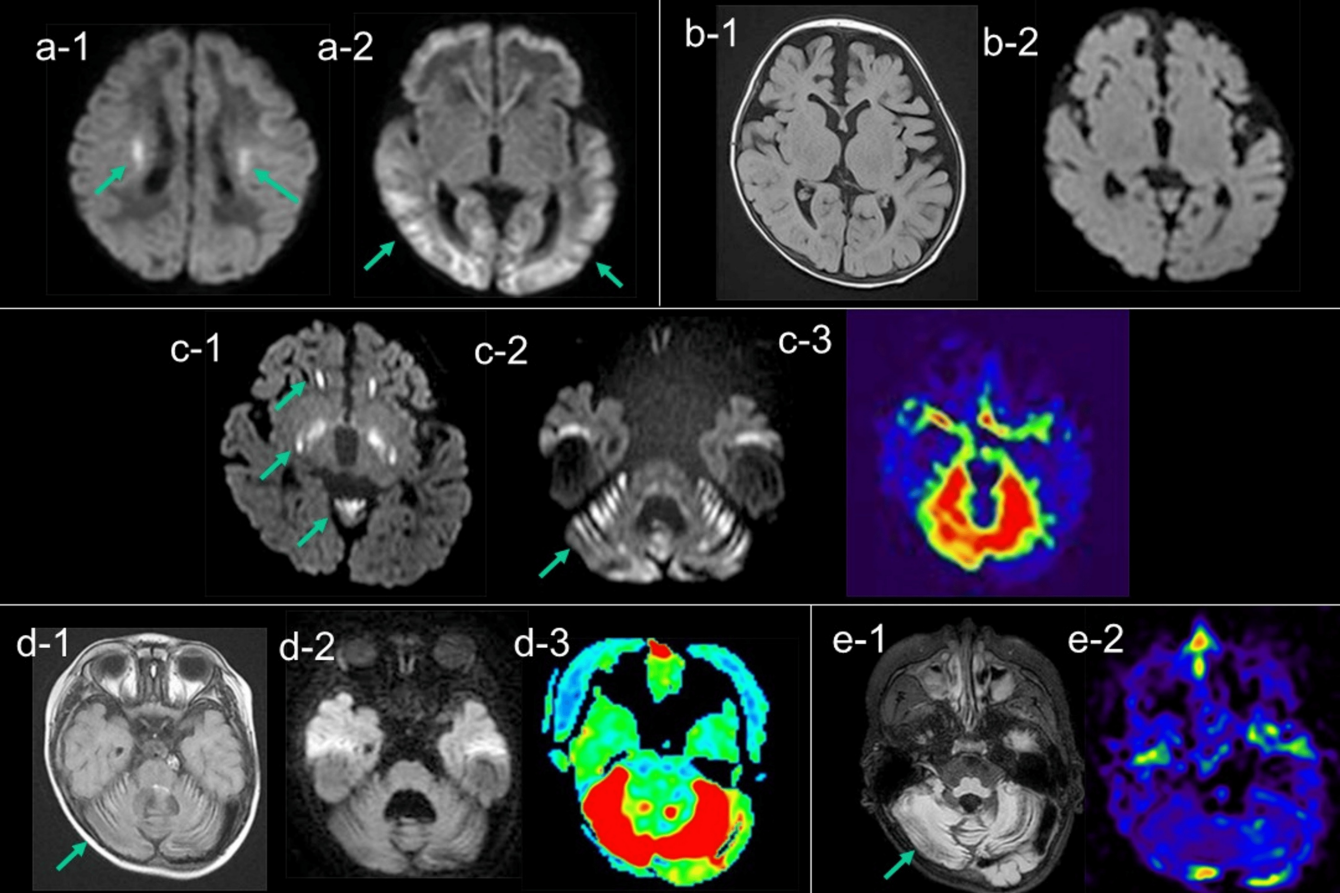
Temporal changes in MRI (a) DWI showed reduced diffusion in the peri-rolandic subcortical white matter (a-1: arrow) on day 3, followed by that in the subcortical white matter on day 7 (a-2: arrow). (b) FLAIR (b-1) and DWI (b-2) at three months revealed mild cerebral atrophy without obvious parenchymal signal abnormalities. (c) At one year and one month, DWI showed reduced diffusion in the globus pallidus, substantia nigra, anterior periventricular white matter, and cerebellar cortex (c-1 and c-2: arrow). ASL showed hyperperfusion in the cerebellum (c-3). (d) At two years and two months, FLAIR showed high signal intensity in the atrophic cerebellar cortex (d-1: arrow). DWI showed no diffusion abnormalities (d-2); however, ASL still showed hyperperfusion in the cerebellum (d-3). (e) At three years and eight months, FLAIR showed progressive cerebellar atrophy (e-1: arrow) with hypoperfusion on ASL (e-2). MRI: magnetic resonance imaging; DWI: diffusion-weighted imaging; FLAIR: fluid-attenuated inversion recovery; ASL: arterial spin labeling
